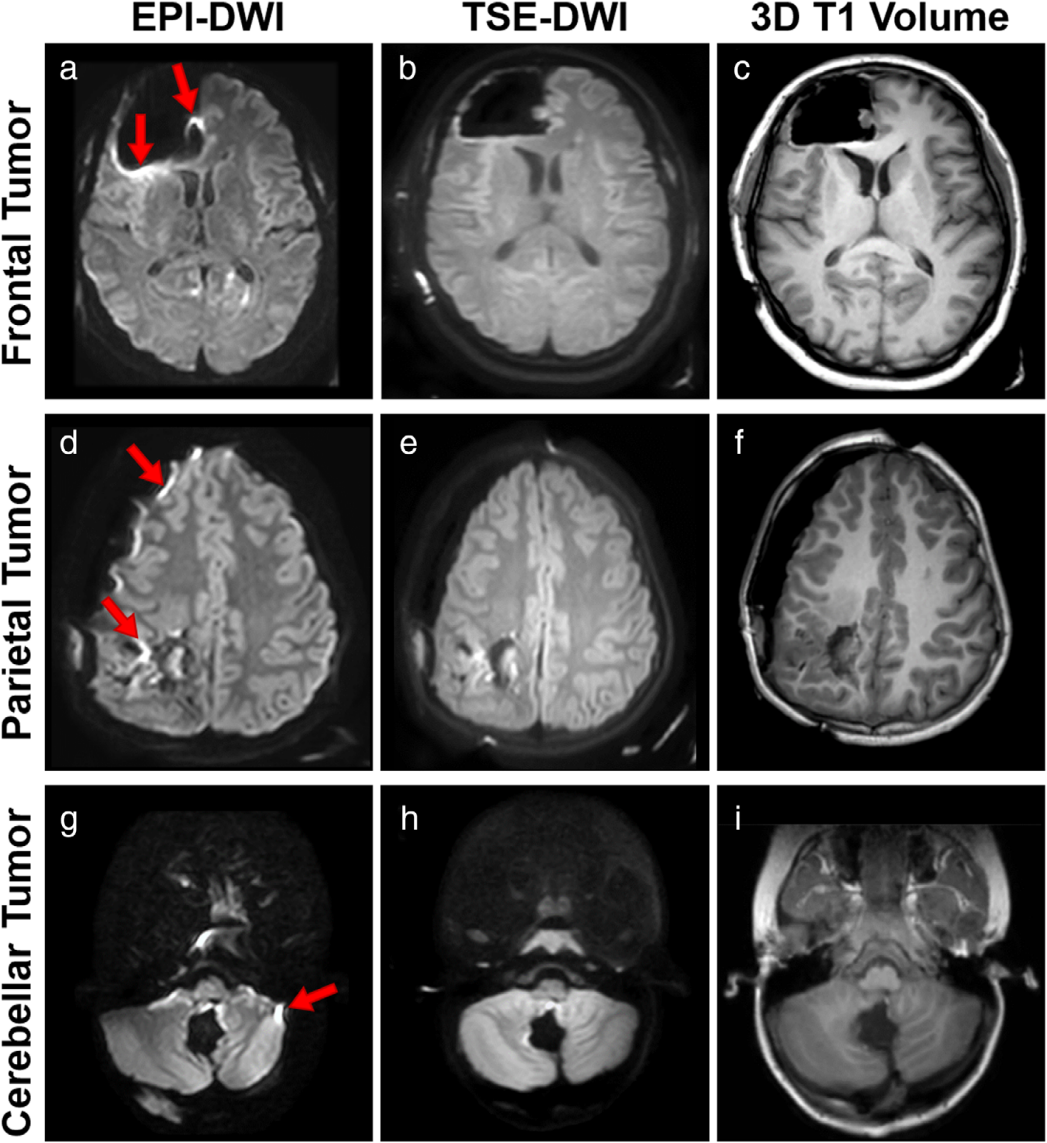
Comparison between b = 1000 EPI‐DWI (a, d, and g), b = 1000 TSE‐DWI (b, e, h), and 3D T1 volume (c, f, i) of IoMRI patients with frontal lobe (a–c), parietal (d, e, f) and cerebellar (g, h, i) resection with signal pile up artifact highlighted with red arrows.
